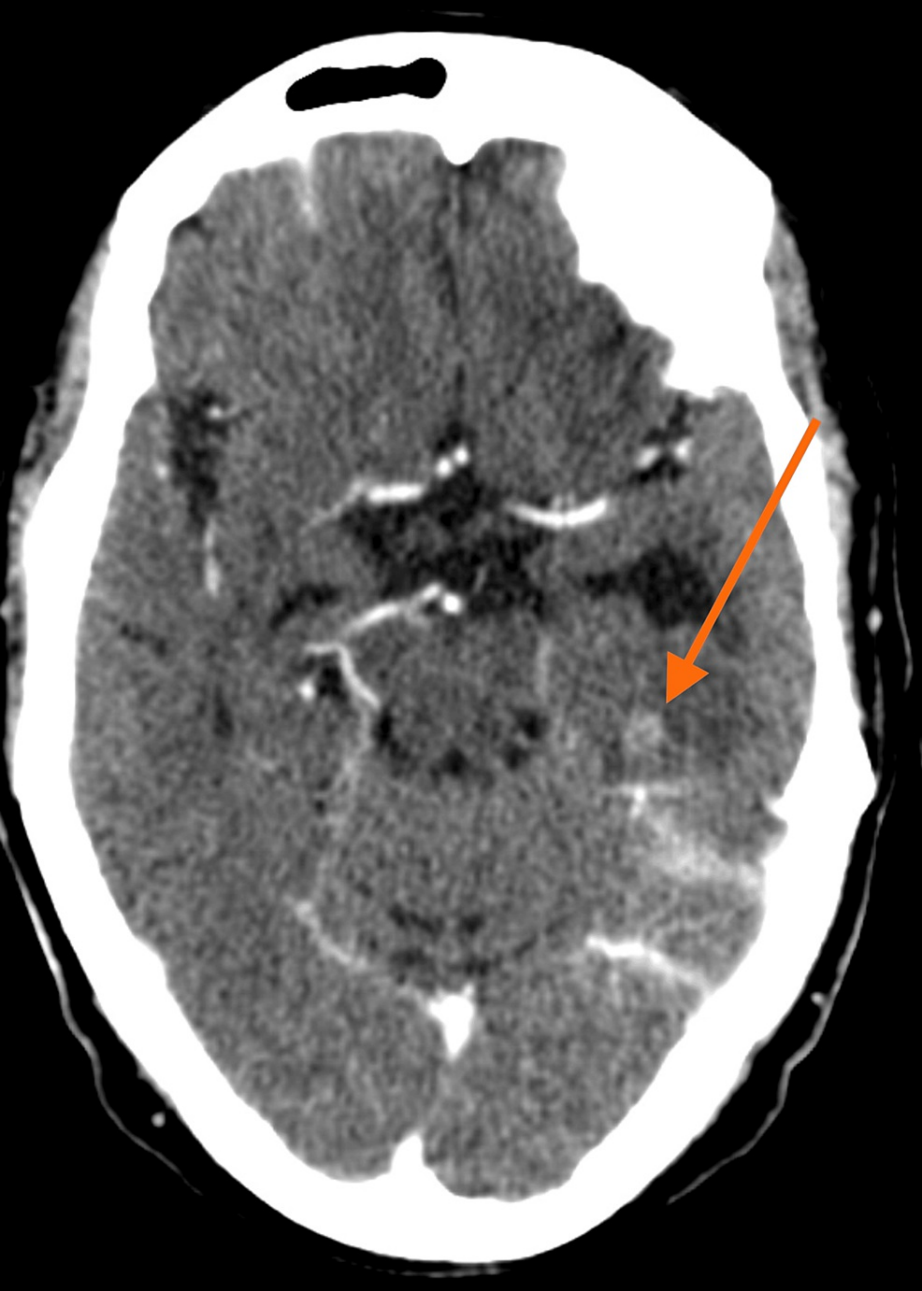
Brain CT shows metastasis in the left temporal lobe.
